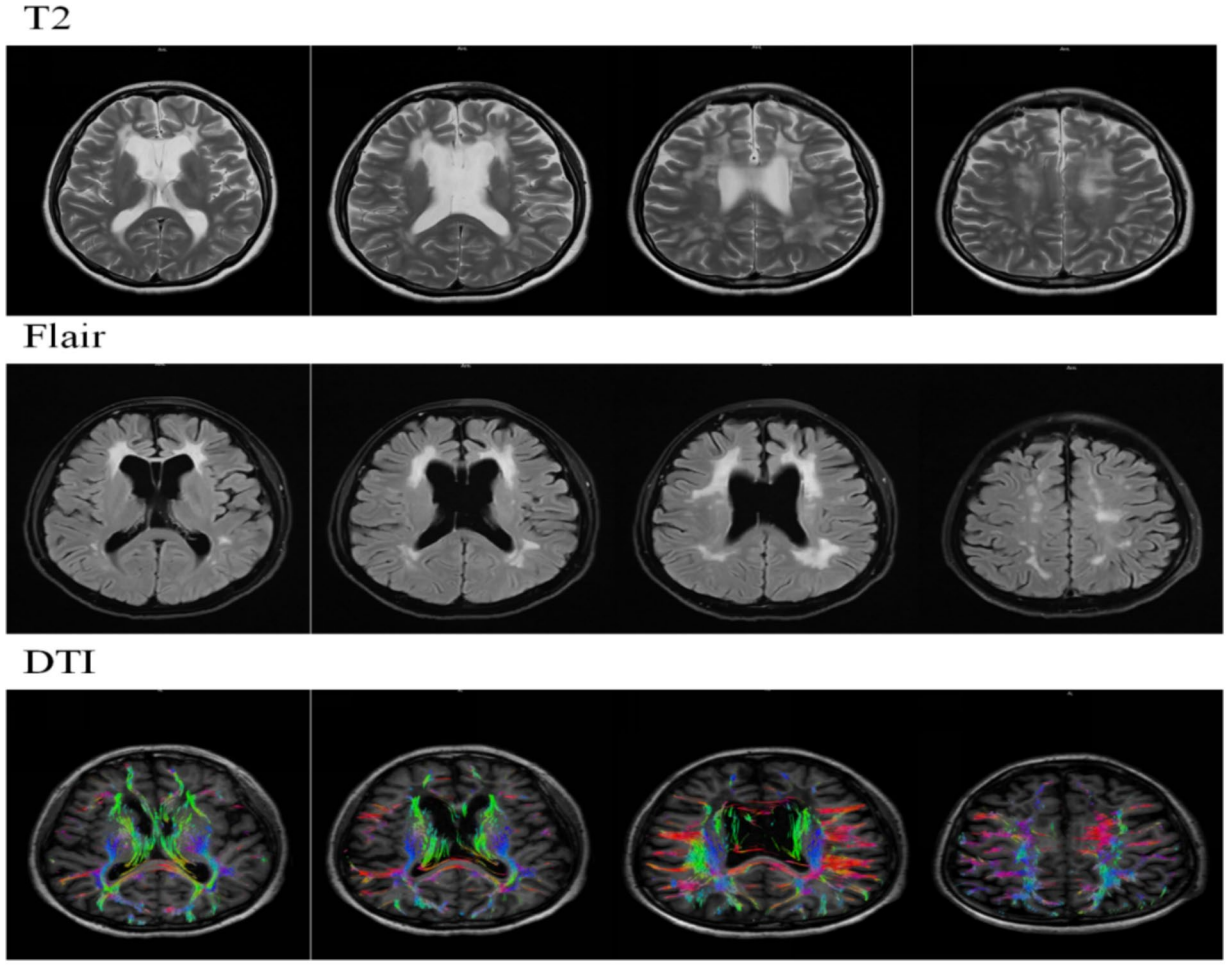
MRI and DTI findings on November 21, 2024.
